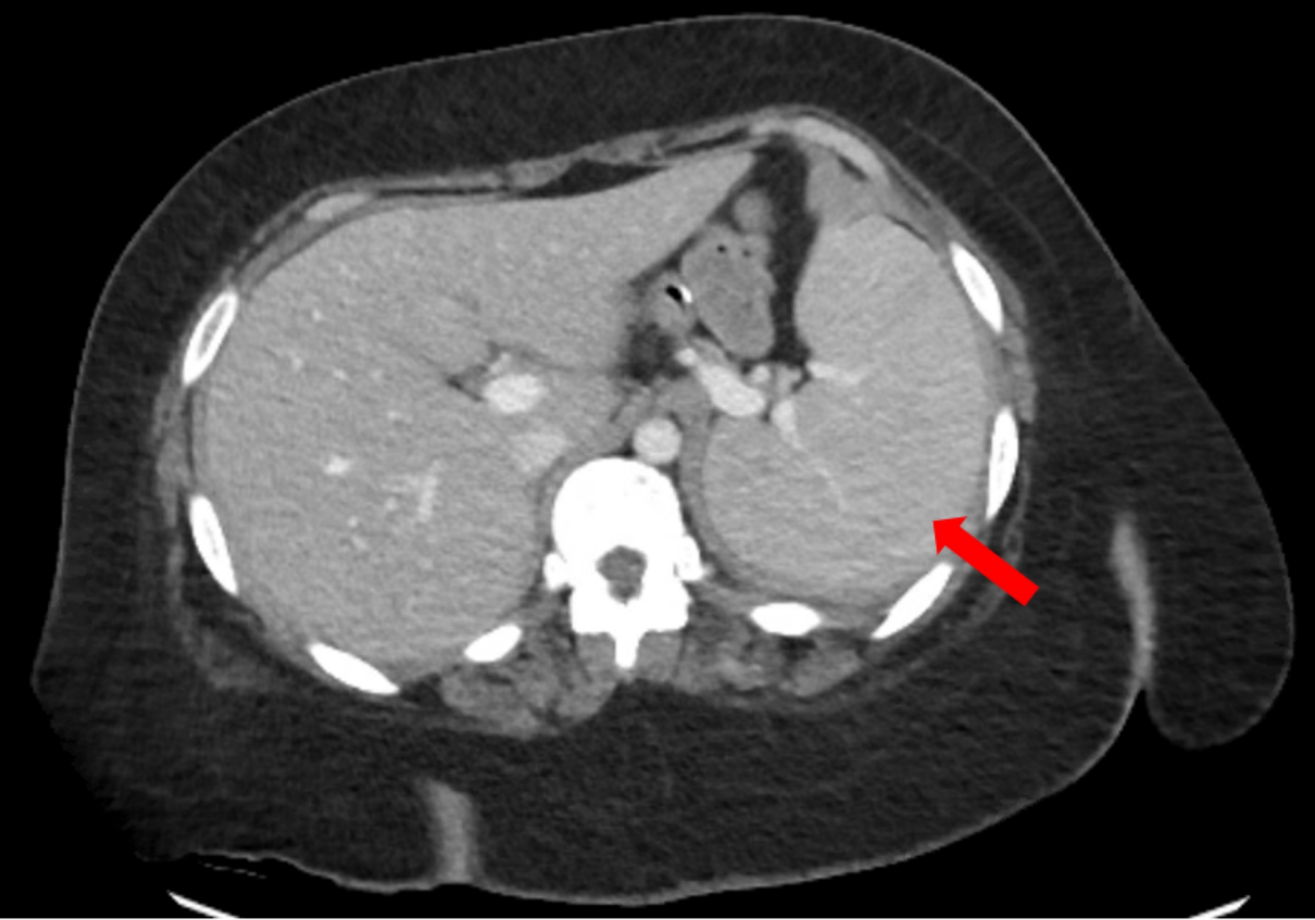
CT scan of the abdomen showing evidence of splenomegaly (red arrow).
